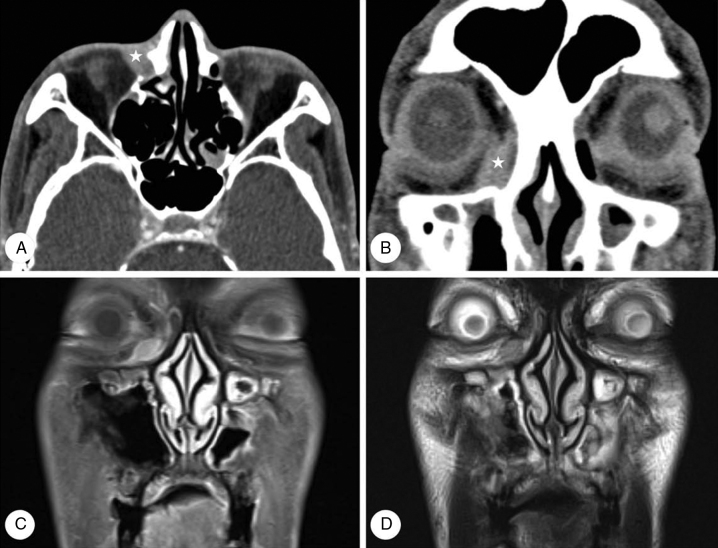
Computed tomography (CT) and magnetic resonance (MR) images of case 1. Axial (A) and coronal (B) CT images reveal a soft tissue mass with indistinct margins on the inferomedial side of the right orbit (). MR images reveal an oval-shaped orbital mass displaying mild enhancement on post-contrast T1 images (C) and intermediate signal intensity on T2 images (D).
